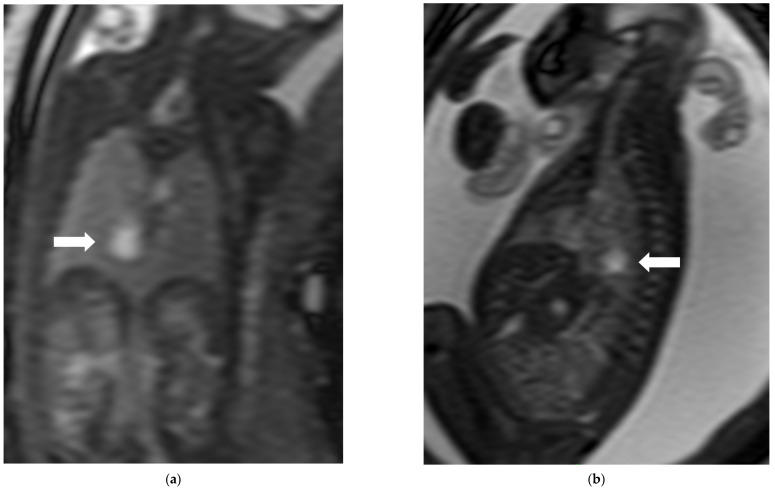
Fetal MRI was performed at 23rd week of gestation for delineation of hyperechoic mass with cystic components in mediastinum. (a) Coronal T2W sequence and (b) sagittal T2W sequence shows a T2W hyperintense cystic lesion in the medial aspect of the right lung adjacent to mediastinum, suggestive of congenital pulmonary airway malformation (CPAM).
